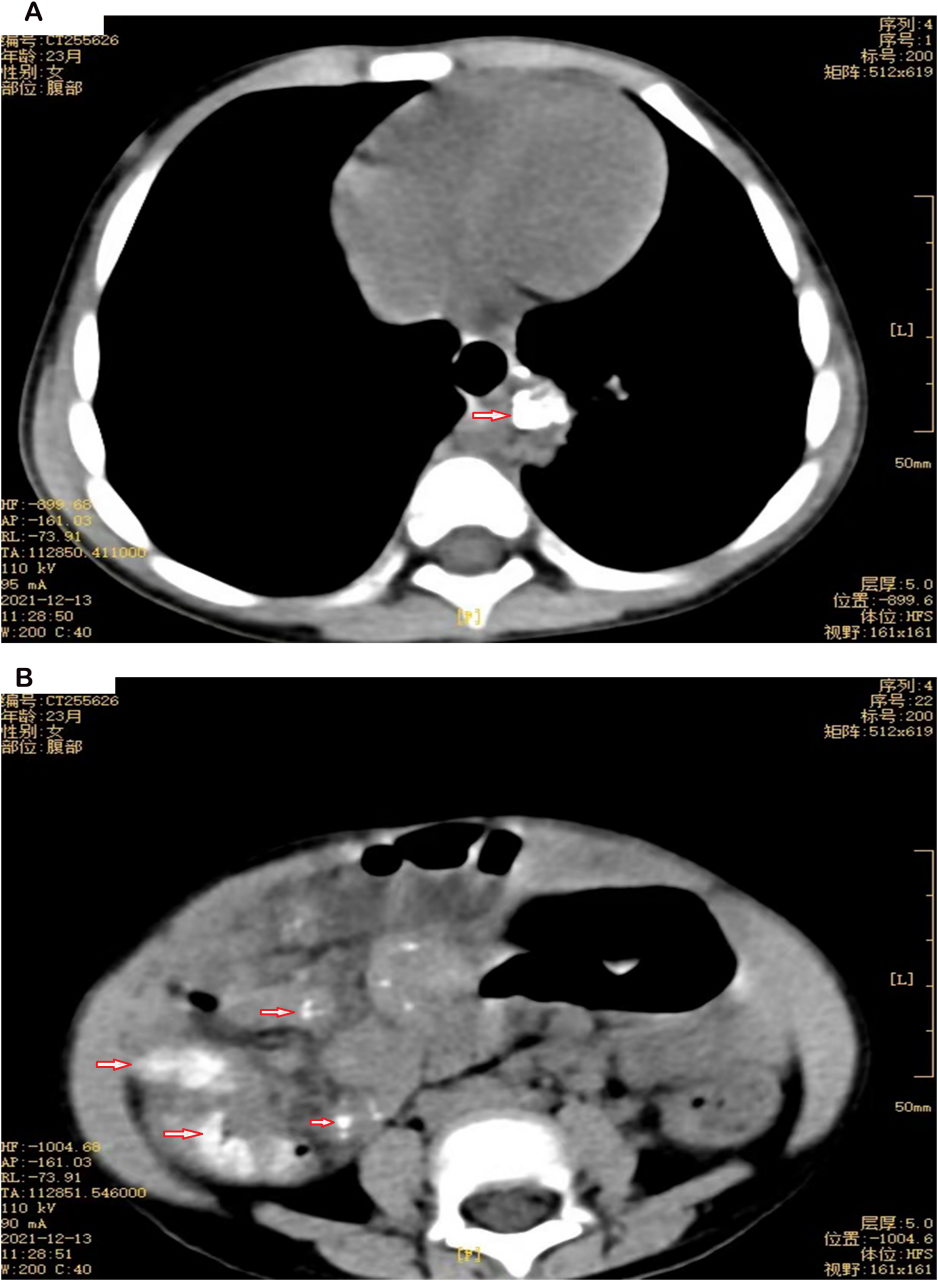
(A) Chest CT of P19, a 23-month-old female. The arrow points to soft tissue in the left posterior lower mediastinum with calcification. (B) Abdominal CT of P19, a 23-month-old female. The arrow indicates multiple nodules with calcification in the abdominal and mesenteric space.
